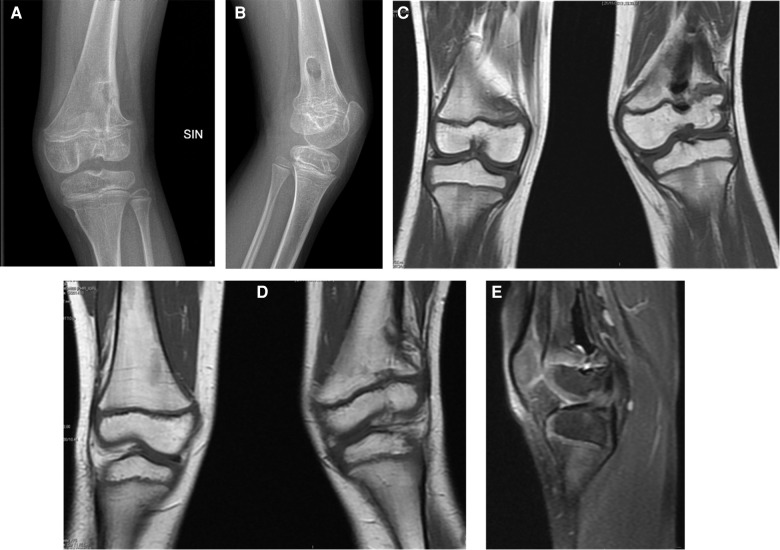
X-ray (A,B) and MRI (C–E) highlighted the results of the recent de-epiphysiodesis operation with placement of bone wax and minimal residual bone bridge.
